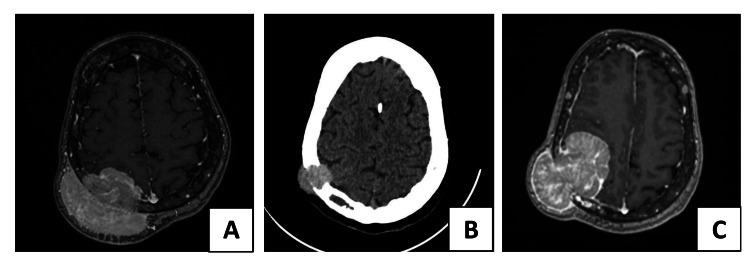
(A) Axial brain MRI at the time of presentation showing an exophytic, hyperintense mass extending intra- and extracranially. (B) Axial brain CT at the largest diameter of the tumor after spontaneous regression. (C) Brain MRI showing re-progression of the hyperintense tumor with greater intracranial invasion. By comparing the initial MRI (A) and the last MRI (C), there is clear regression of the extracranial component of the lesion; however, there is extensive progression of the intracranial component, which explains the patient’s clinical manifestations.
